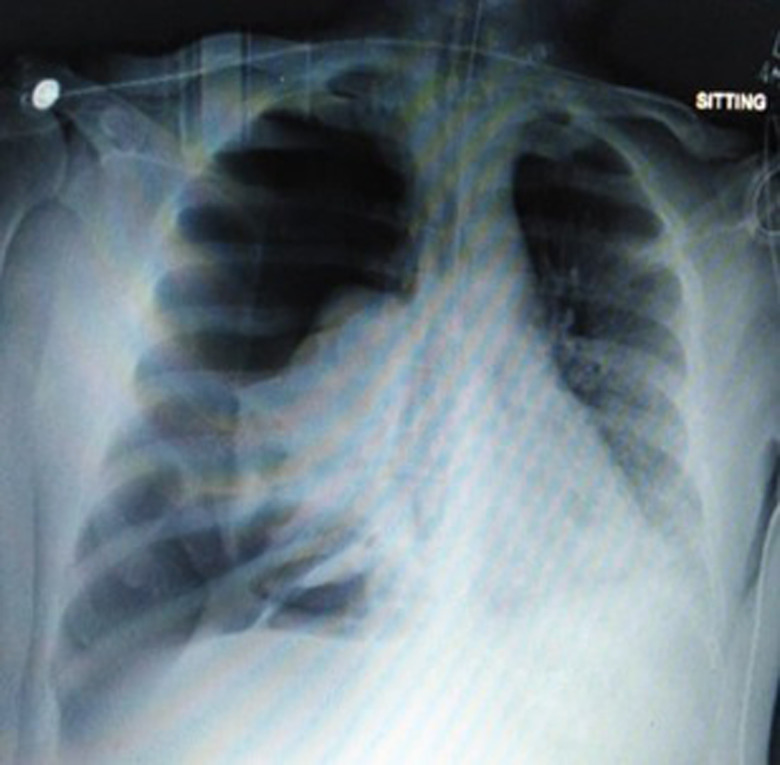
Chest x-ray showing right pneumothorax.
